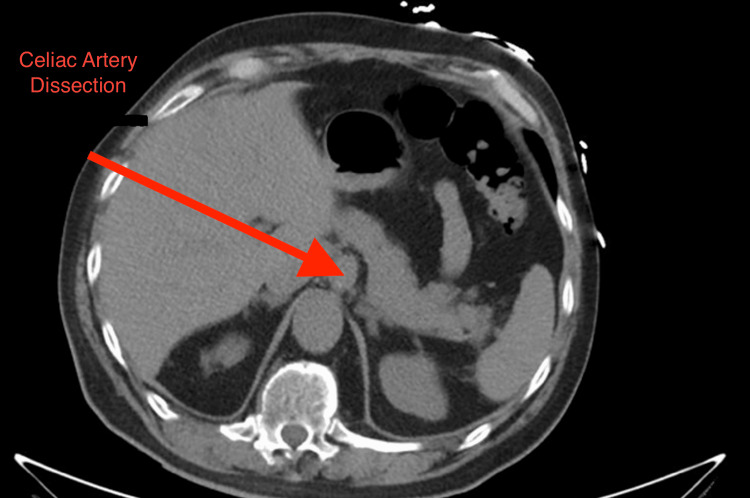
Contrast-enhanced axial CT abdomen The contrast-enhanced axial CT image of the abdomen demonstrating aneurysmal dilation of the celiac artery (arrow), consistent with focal dissection. The true lumen is narrowed, and there is no evidence of aortic involvement.
